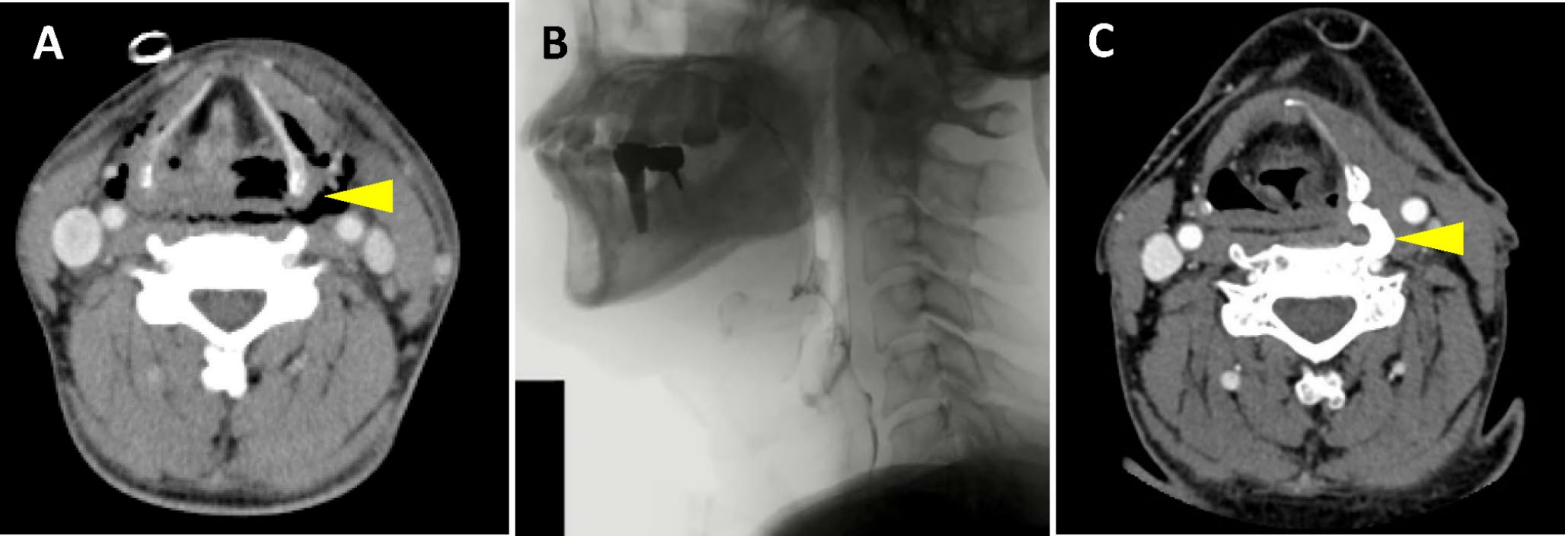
Preoperative examination. A: Contrast-enhanced computed tomography image of the neck at the onset of a deep neck abscess. Extensive neck emphysema was found, predominantly around the left hypopharynx (arrowhead).　B: Preoperative videofluorographic swallowing study. C: Contrast-enhanced neck computed tomography 7 months after onset revealed an abnormal bony outgrowth of the cervical vertebra (arrowhead)
